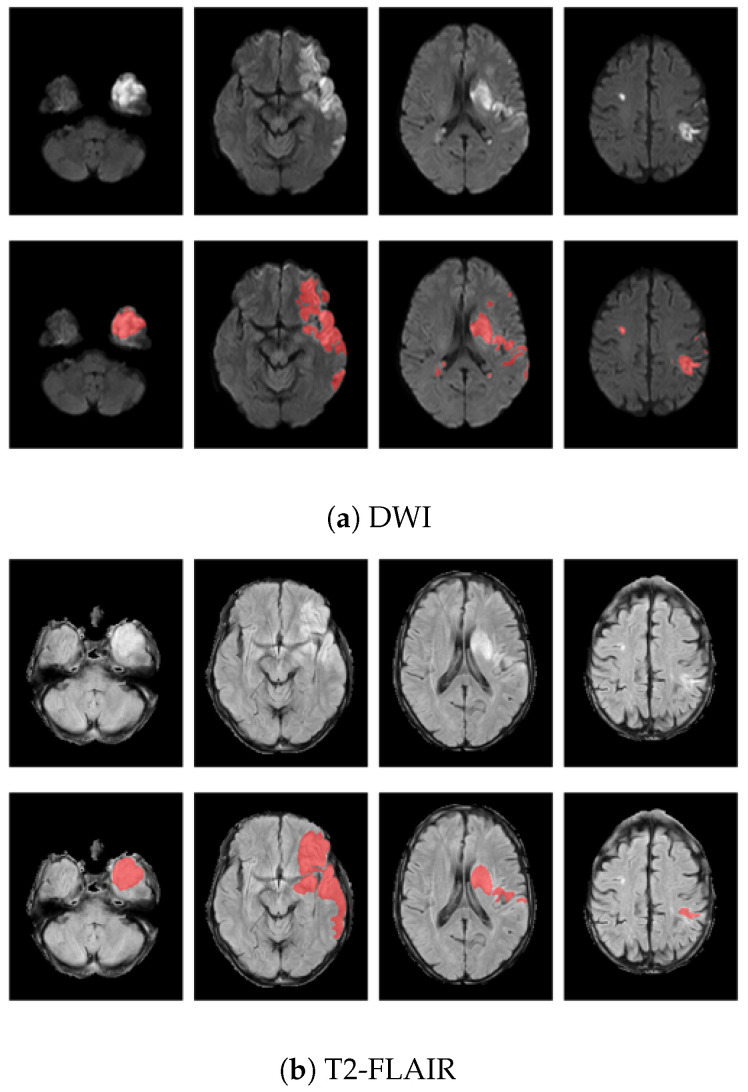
Example of infarct lesion segmentation on a selected slices sampled from (a) DWI and (b) T2-FLAIR preprocessed volumes. For each modality, original slices are displayed in the first row and lesion segmentation (red) is shown as overlapping the correspondent slices in the second row.
